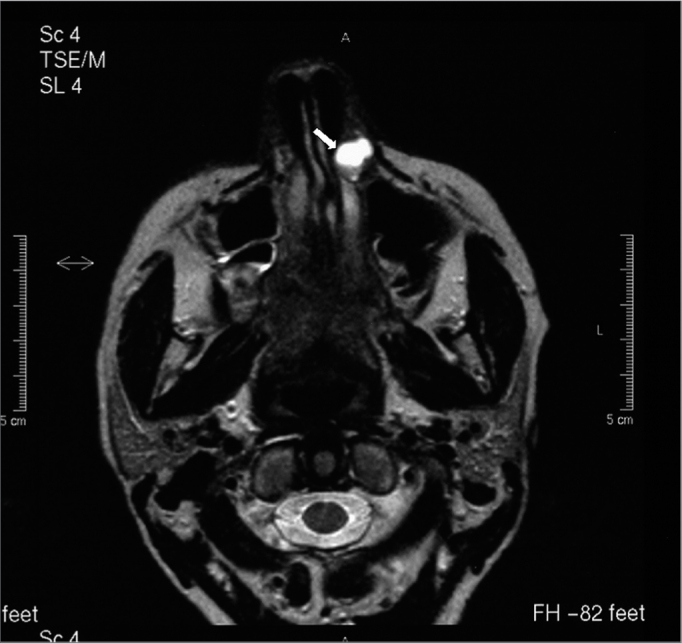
B-) T1-weighted MRI image (axial section). Note that the lesion contains isointense areas, and hyperintense areas in certain regions.
